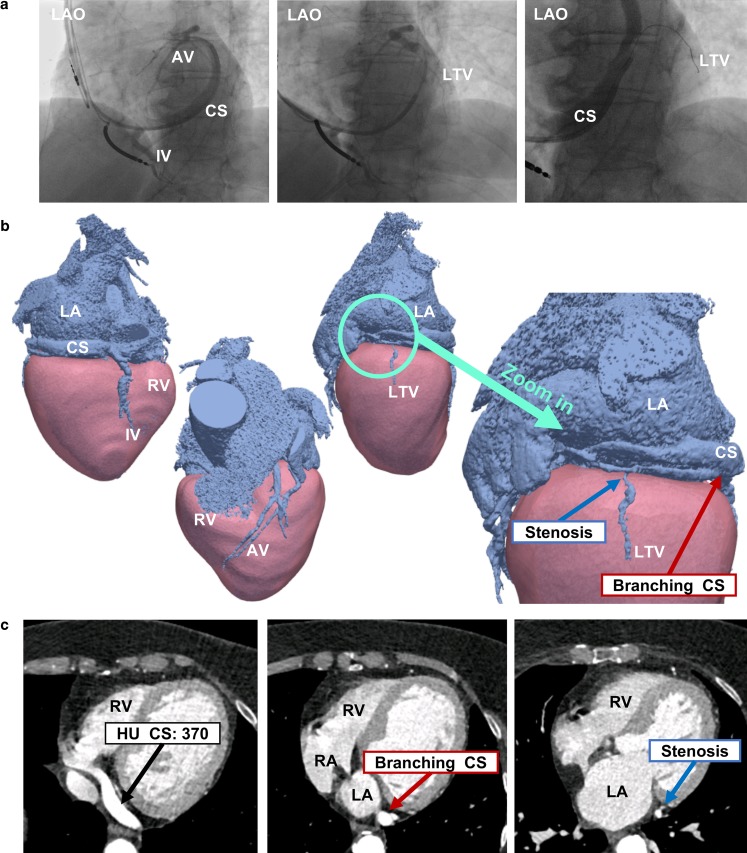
A 78-year-old woman (patient 16) who had undergone an unsuccessful cardiac resynchronisation therapy implantation 5 years earlier as no lateral vein on FA (a) was accessible for left ventricular lead placement. CTA in 3D (b) and 2D (c) revealed a single small and stenotic LTV and CS branching. *AV* anterior vein, *CS* coronary sinus, *IV* inferior vein, *LA* left atrium, *LAO* left anterior oblique, *LTV* lateral vein, *RA* right atrium, *RV* right ventricle
